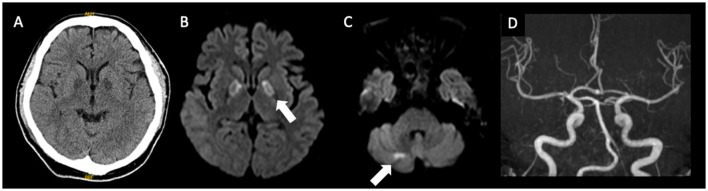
Imaging findings at the initial examination, (A), CT; (B), (C): MRI; (D), MRA. Bilateral involvement of the globus pallidus and abnormalities in the right cerebellar hemisphere are evident. No major vessel stenosis is observed. CT, computed tomography; MRI, magnetic resonance imaging; MRA, magnetic resonance angiography.
